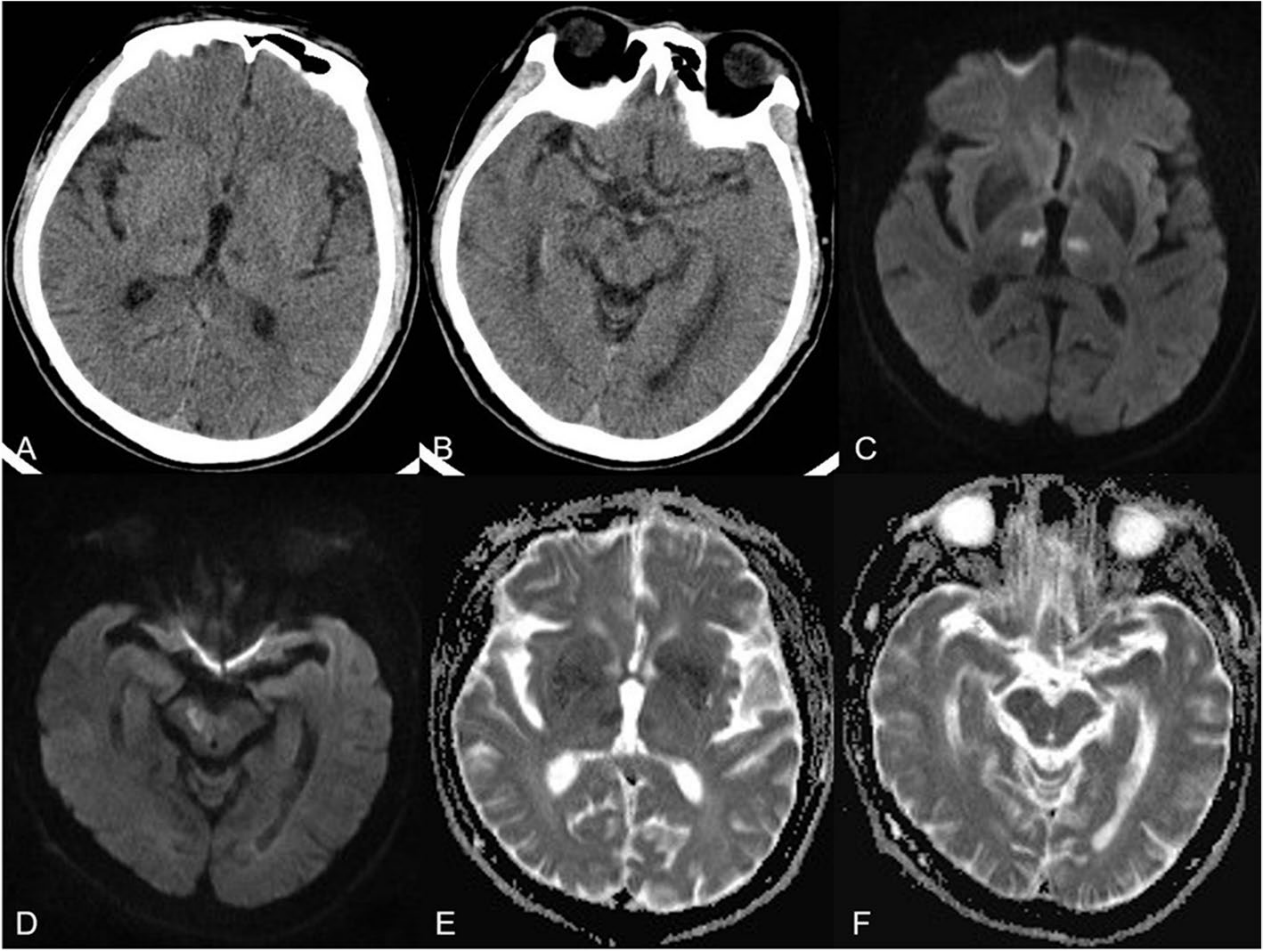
Bilateral parathalamic and rostral midbrain hypodensity on axial head CT. Case 2. Axial head CT at 2 hours after onset (A and B) shows hypodensity in the bilateral paramedian thalamus and midbrain involvement (white arrow). Axial DWI and ADC maps (C to F) show acute symmetrical infarctions in the bilateral paramedian thalamus and show a V-shaped acute infarction at the interpeduncular fossa of midbrain
